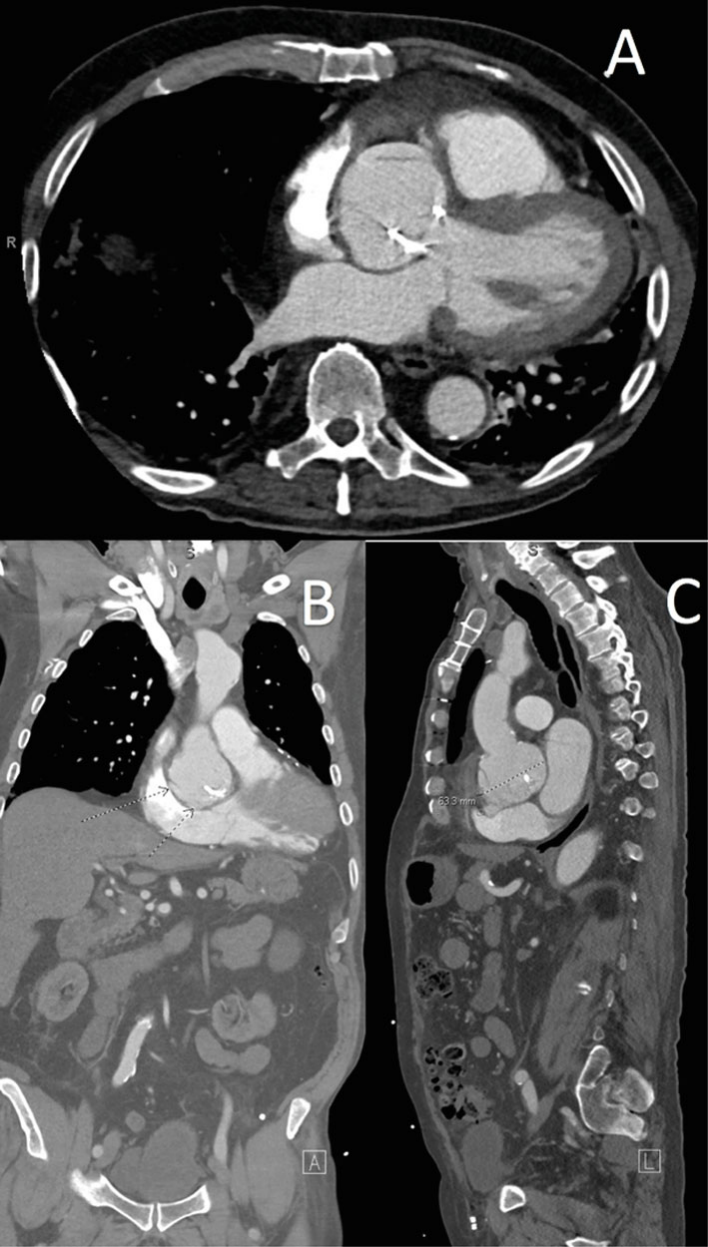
(A–C) Computed tomography angiography of the chest. Demonstration of a 6.7-cm aneurysmal dilation of the aortic root with a thin dissection and active contrast extravasation into the right atrium.
